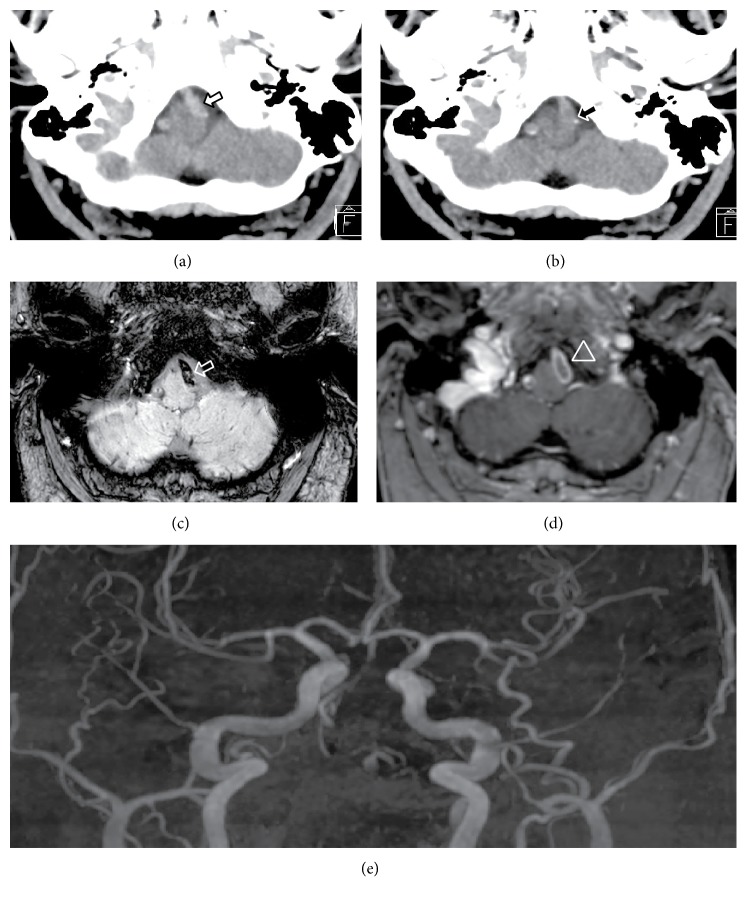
Seven months before presentation left VA is patent with intraluminal average Hounsfield units (aHU) of 60.6 on native CT (open black arrow) (a). 23 hours after symptom onset. Black arrow shows subtle hypodensity with aHU of 42.4 in the left VA (closed white arrow) (b). Three days after symptom onset susceptibility weighted effect in left VA (empty white arrow) (c) and flow void with rest-flow around clot in contrast-enhanced MR T1 (open white arrowhead) (d). MR time-of-flight angiography showing the absence of flow in both VAs (e).
